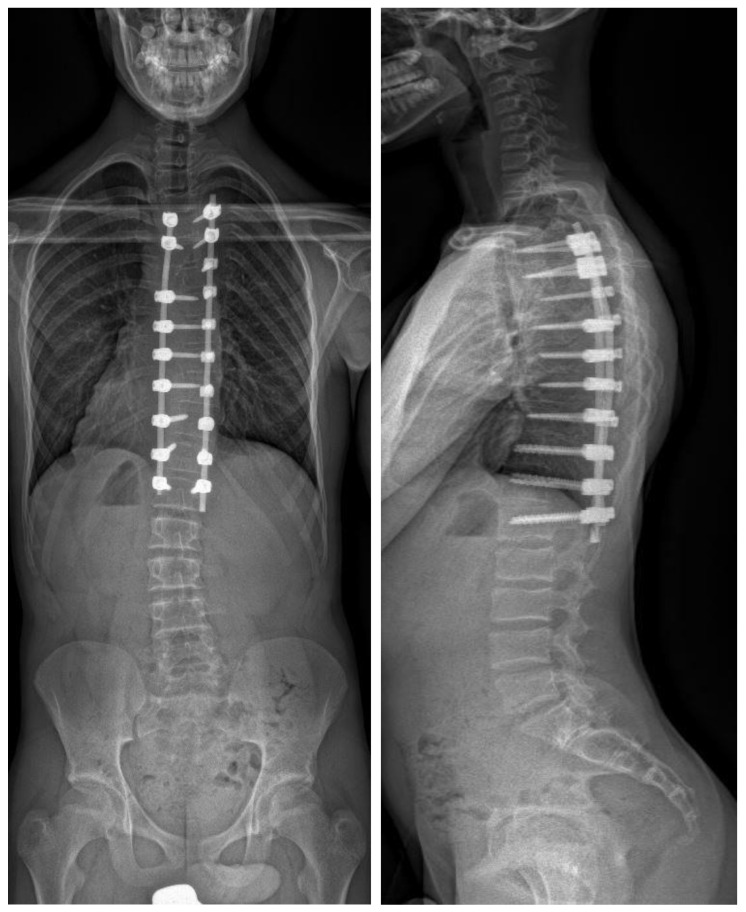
Biplanar radiograph of the same patient after posterior instrumented fusion using freehand technique. The intraoperative DAP was 638.2 mGy/cm^2^.
